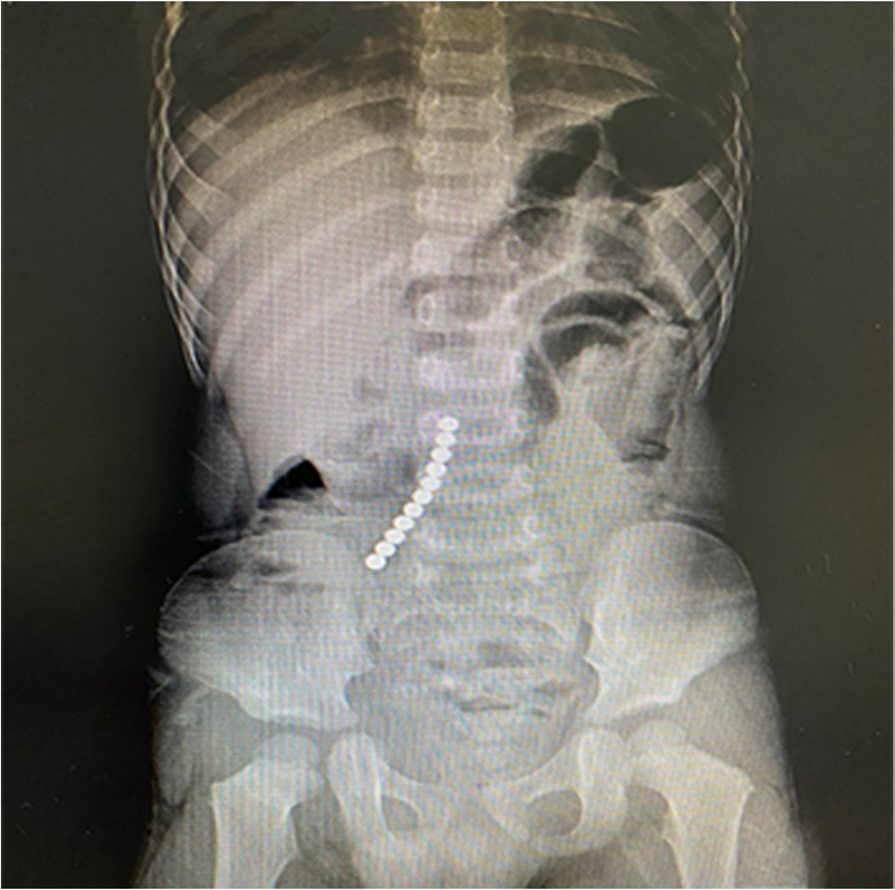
The x-ray showed that the foreign bodies were in the middle and lower abdomen. The patient had obvious symptoms of abdominal pain and vomiting. After laparotomy, it was found that the foreign bodies were in the stomach and small intestine, which attracted each other and caused injury
